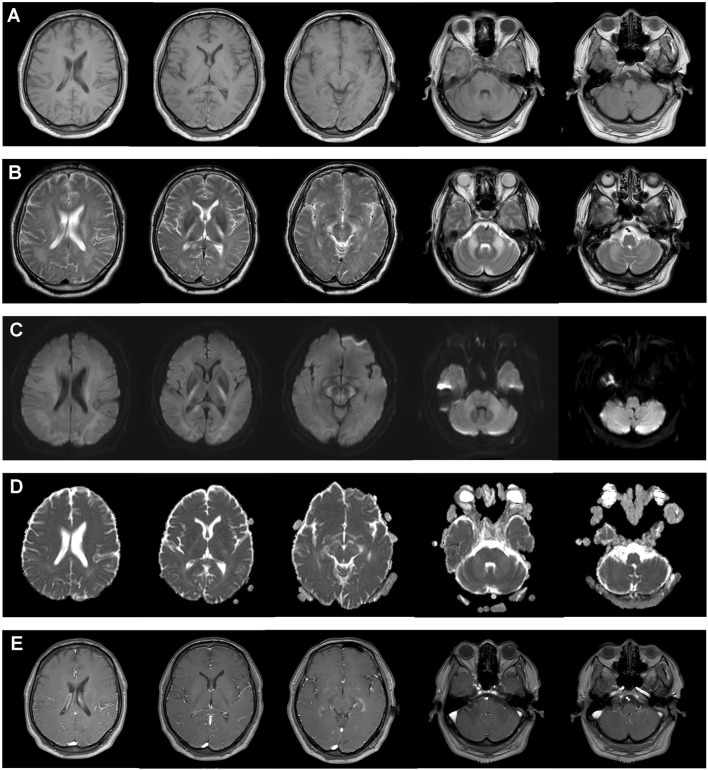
Brain magnetic resonance imaging of the patient. Symmetrical and confluent white matter abnormalities with hypointense signals on T1-weighted images (A) and hyperintense signals on T2-weighted images (B) were observed in the posterior limbs of the internal capsules; midbrain cerebral peduncles; middle cerebellar peduncles; bilateral frontal, parietal, and occipital white matter; the splenium of the corpus callosum; and cerebellar white matter. High signal intensity on diffusion-weighted imaging (C) without restriction on the apparent diffusion coefficient (D) was observed in the involved areas. (E) No contrast enhancement was observed in the post-gadolinium T1-WI sequence.
